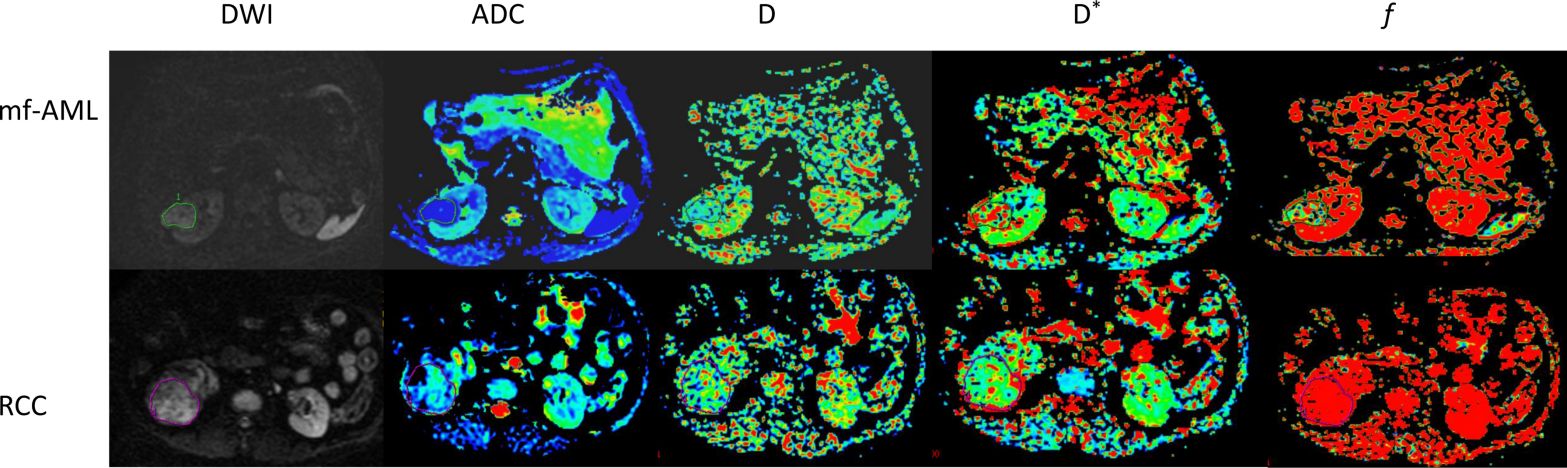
IVIM-DWI images of mf-AML and RCC. The tumors were displayed in DWI images. The D, D*, and *f* maps were obtained from IVIM of mf-AML in the top low and RCC in the bottow row, respectively. Outlines indicate the tumor region.
